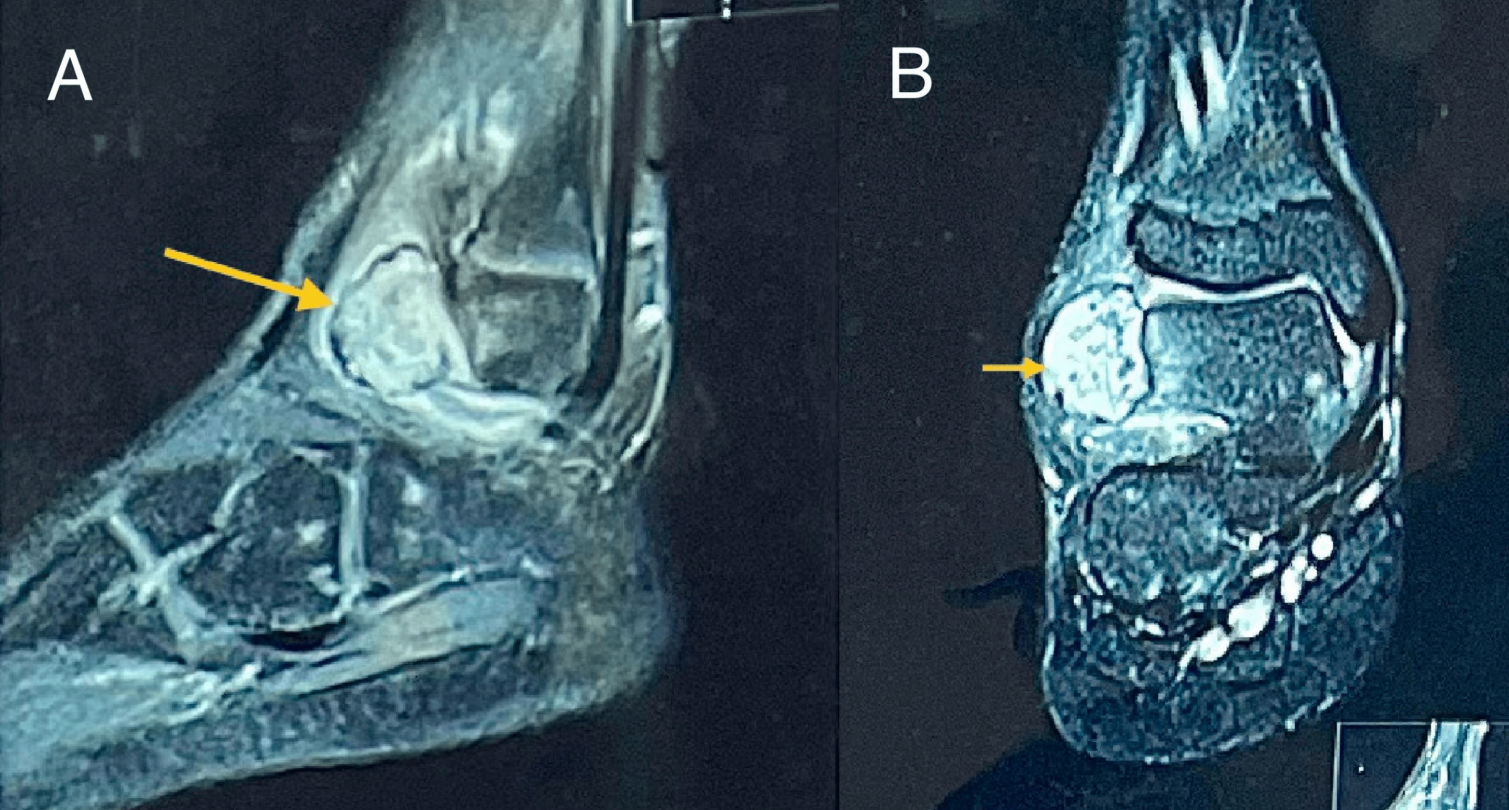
MRI with saggital (A) and coronal (B) sections showing a well-defined soft-tissue intensity lesion in the subcutaneous plane over the ankle joint anterior to the lateral malleolus. The lesion appears to be abutting the lateral aspect of the talus and causing scalloping of its lateral cortex. Adjacent bone marrow edema can be seen on PDFS sequences. PDFS: proton density with fat suppression
